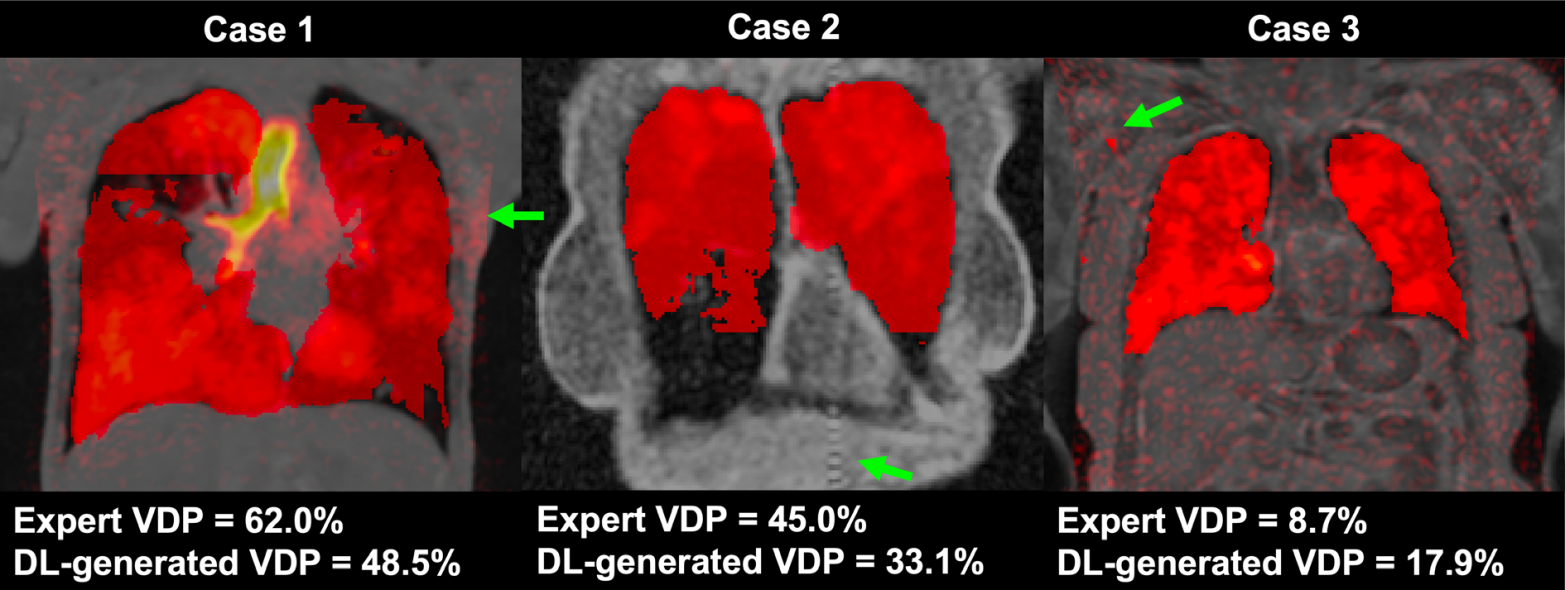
Example coronal slices of fused ^1^H‐MRI, ^129^Xe‐MRI and LCEs from three cases in the testing set which, through Bland–Altman analysis, fall outside the limits of agreement. Manual and DL‐generated VDPs are given. Case 1 contains a motion artifact on the ^129^Xe‐MRI. Case 2 contains a zipper artifact on the ^1^H‐MRI. Case 3 exhibits a large degree of noise throughout the ^129^Xe‐MRI. Artifacts are indicated with green arrows.
